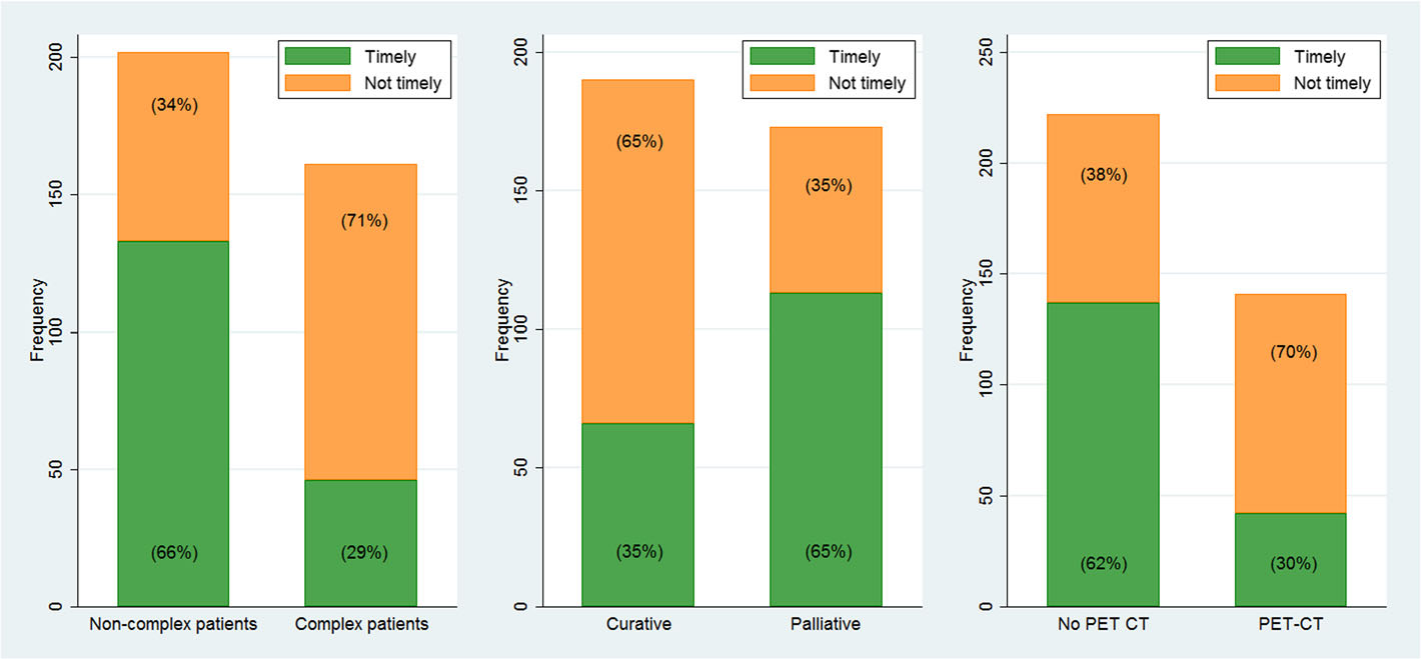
Proportions that received timely lung cancer treatment. Distributions of timely and not timely treatment in non-complex versus complex, curative versus palliative treatment, and having a PET CT versus no PET CT
